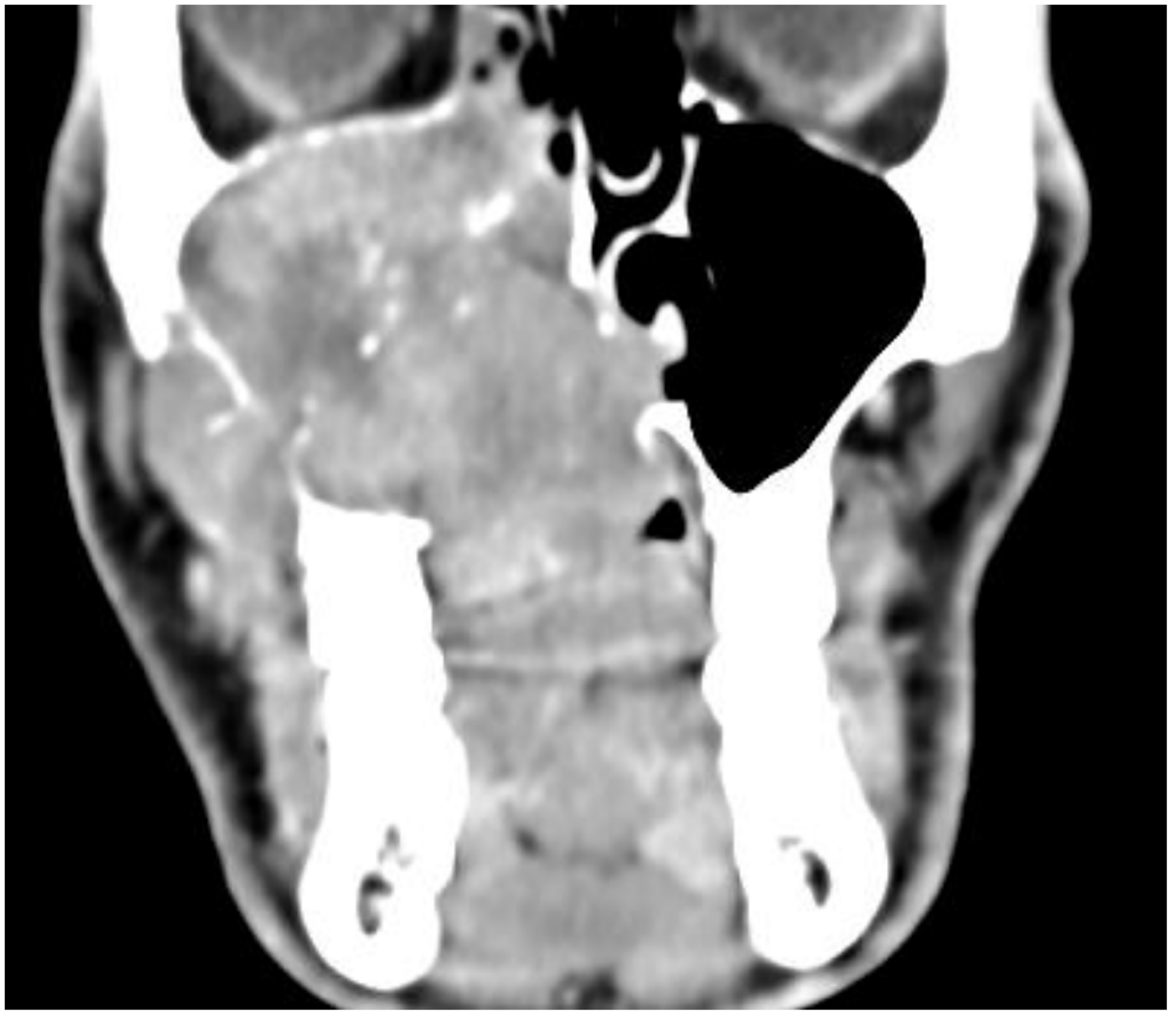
CT-scan in a coronal section showing an adenoid cystic carcinoma as an inhomogeneous mass in the right maxillary sinus with infiltration of neighboring structures.
